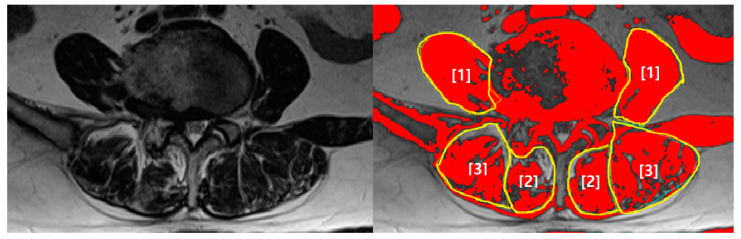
Measurement of CSAs and FCSAs on a representative T2-weighted magnetic resonance (MR) image. (Left): original T2-weighted MR image. (Right): T2-weighted MR image showing CSAs as the area surrounded by the yellow lines and FCSAs as the red colored areas. [1]: Psoas, [2]: Multifidus, [3]: Erector spinae; CSA, cross-sectional area; FCSA, functional cross-sectional area.
